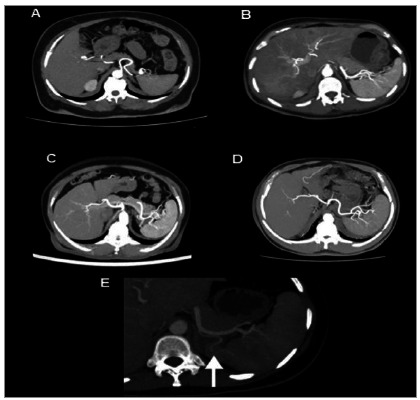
Maximum Intensity Projection (MIP) reconstructions in the axial computed tomography plane demonstrating (A) two terminal branches, (B) three terminal branches, (C) four terminal branches, (D) five terminal branches, and (E) polar artery (white arrow).
